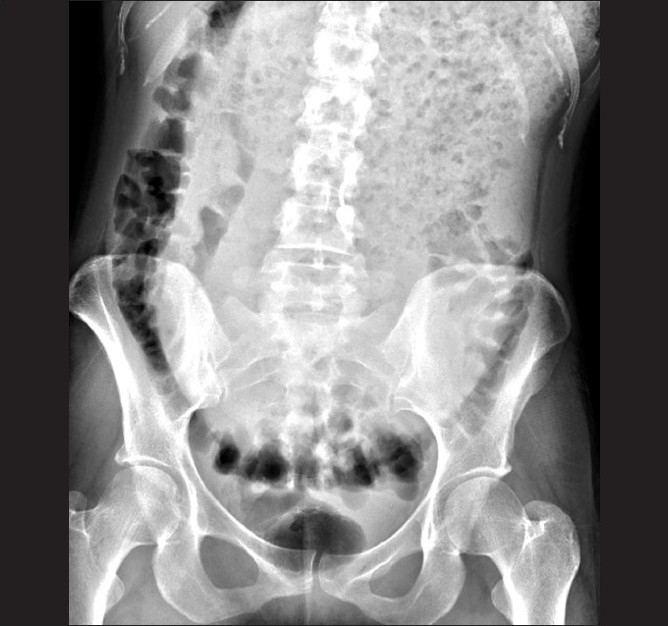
Abdominal radiograph showing a massively enlarged stomach, which occupies the entire abdominal cavity and displaces the transverse colon into the pelvis
